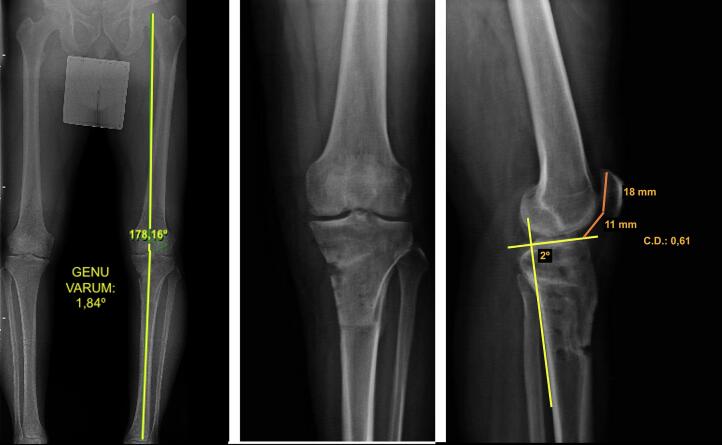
Final postoperative measurements: A) Long-leg alignment radiograph showing correction of varus deformity. B) Monopodal anteroposterior weight-bearing radiograph. C) Measurement of posterior tibial slope and Caton-Deschamps index.
